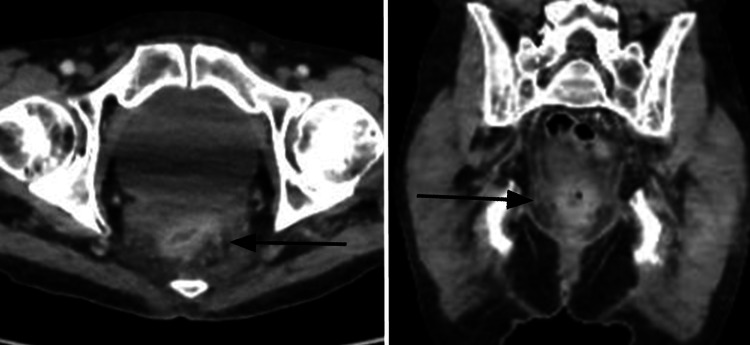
Axial and coronal reconstructions of a CT exam centred on the pelvis showing thickening of the rectal tissue, inflammatory infiltration of the perirectal fat and associated mucosal hyperaemia (black arrows). This is suggestive of a pathology of inflammatory and not oncological origin. CT: computed tomography
